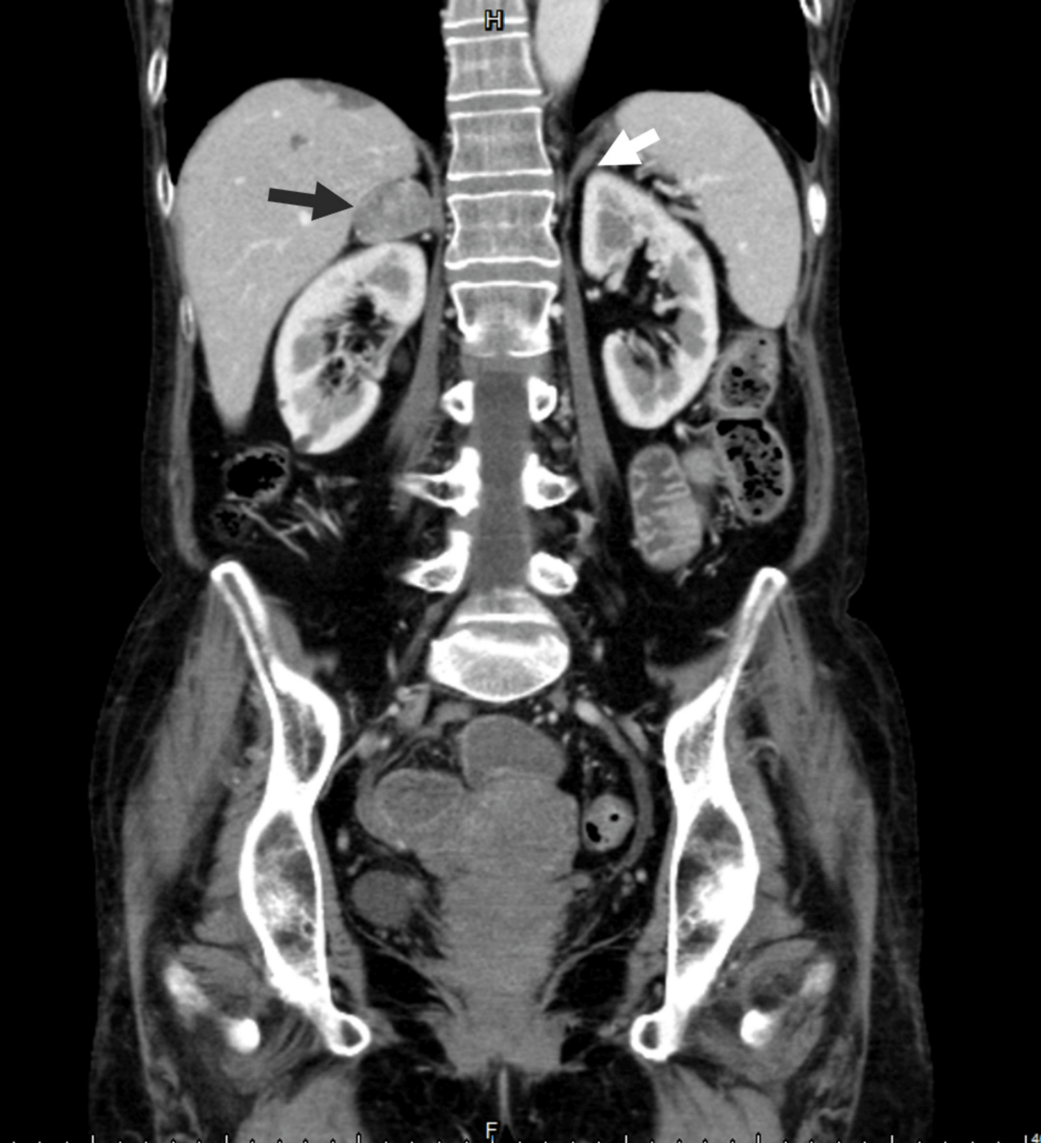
Contrast-enhanced abdominal computed tomography done during this visit (A) Black arrow, a 2.9 cm cyst-like, unenhanced nodule in the right adrenal gland. (B) White arrow, absence of the left adrenal gland due to the prior adrenalectomy.
